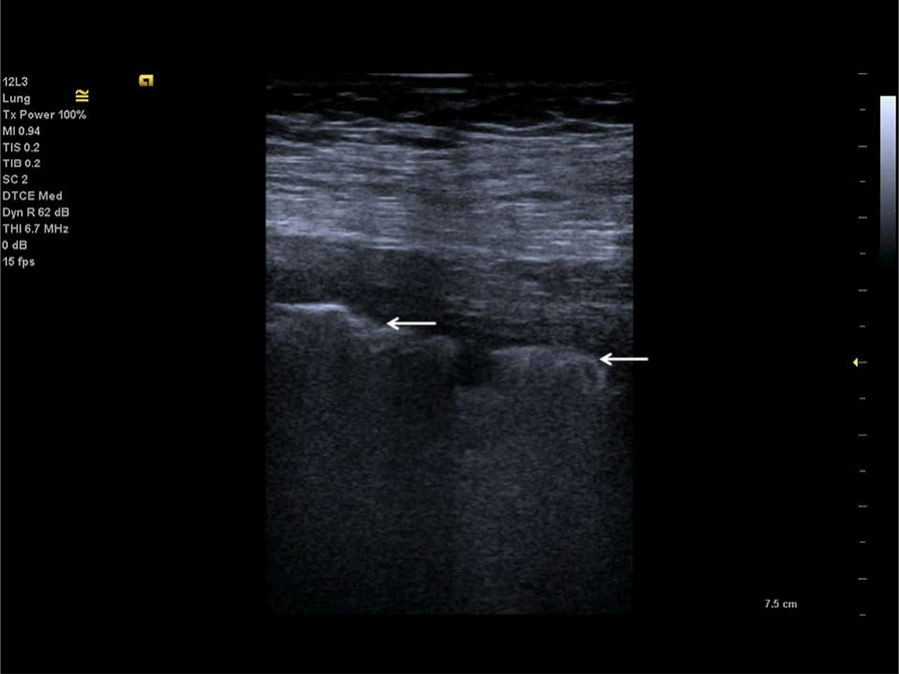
Lung ultrasonography using a linear probe. Discontinuous pleura (white arrows) with tiny round hypoechoic consolidations
